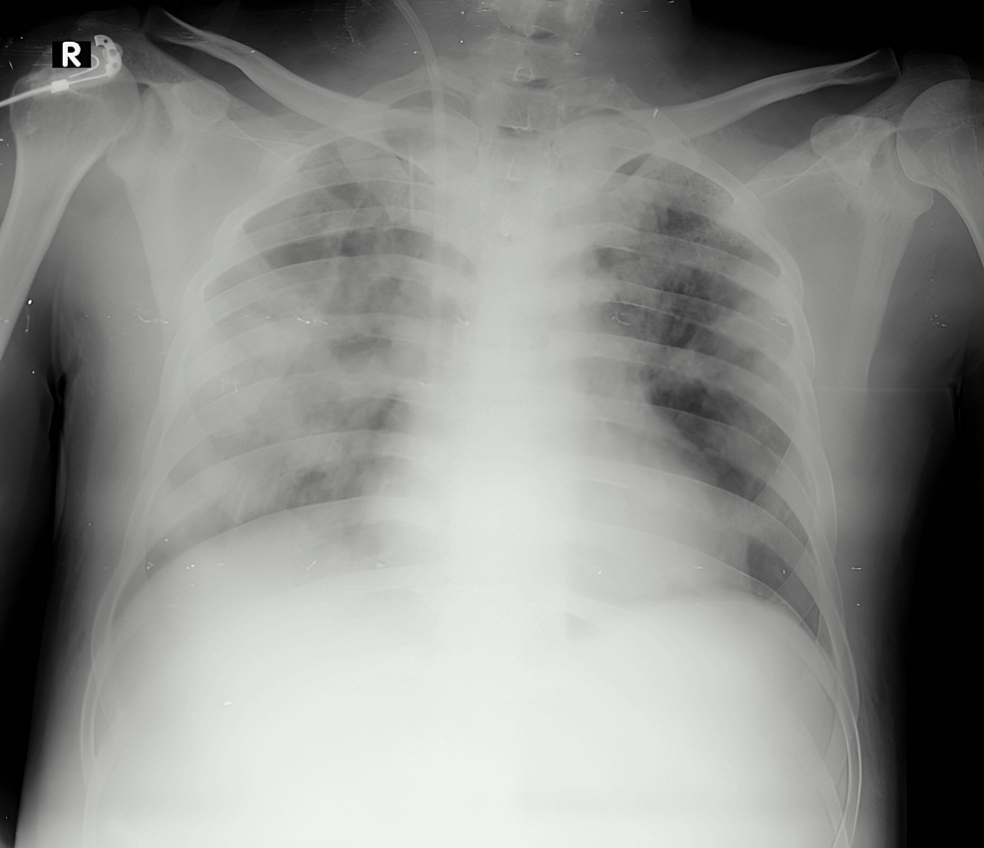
Chest X-ray showing multilobar consolidation
